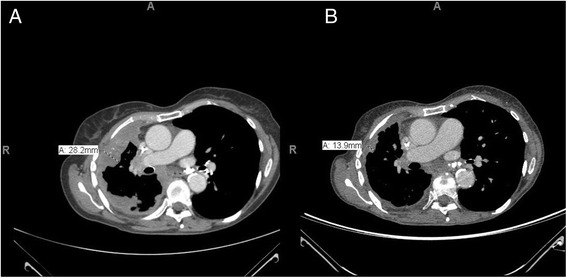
Chest CT Scans of Patient on Crizotinib Treatment. a Chest/Upper Abdomen CT Scan Prior to Crizotinib Treatment. b Chest CT Scan Post-Crizotinib Treatment
